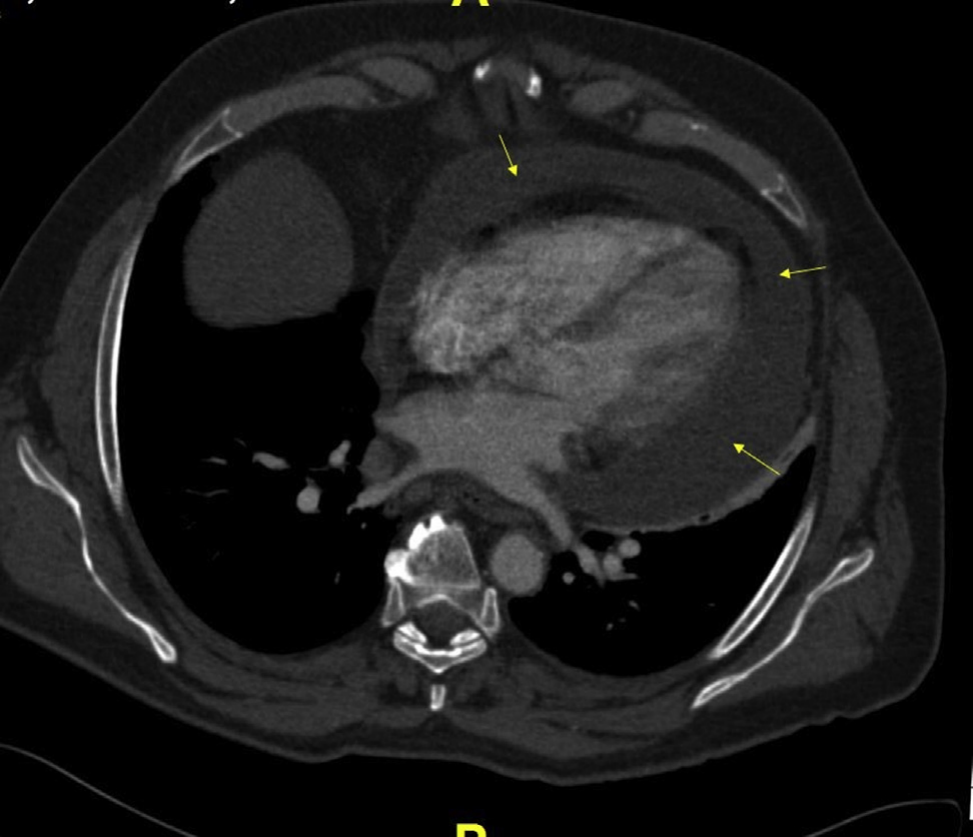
Chest CT scan axial view showing moderate-to-large pericardial effusion (*yellow arrows*).
